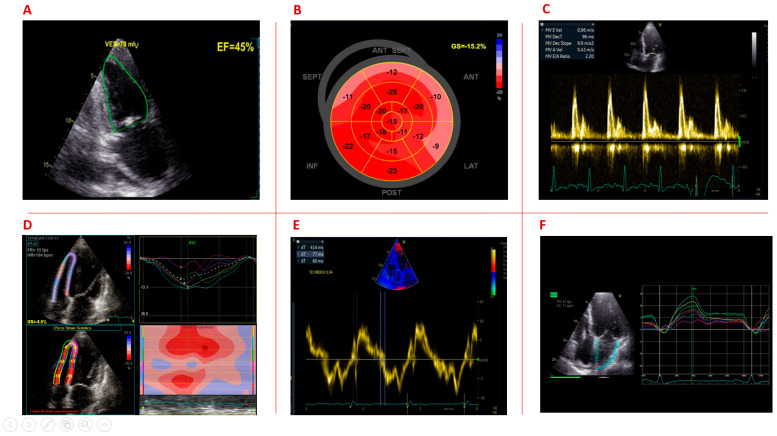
Overview of the echocardiographic functional abnormalities in DM1 patients. (A) Reduced left ventricular ejection fraction; (B) impaired left ventricular global longitudinal strain; (C) diastolic dysfunction; (D) impaired right ventricular longitudinal strain; (E) increased atrial electromechanical delay; (F) impaired left atrial strain.
